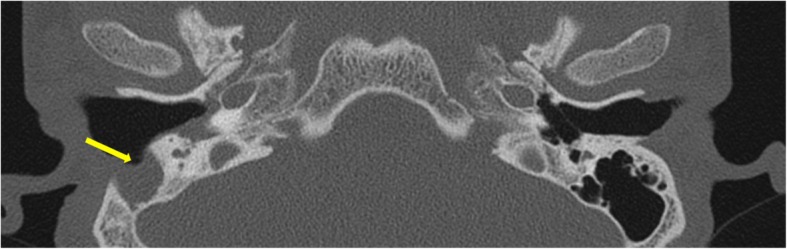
Axial CT shows the right mastoid cavity filled with soft tissue and is seen to open directly into the external auditory canal suggesting automastoidectomy (arrow)
